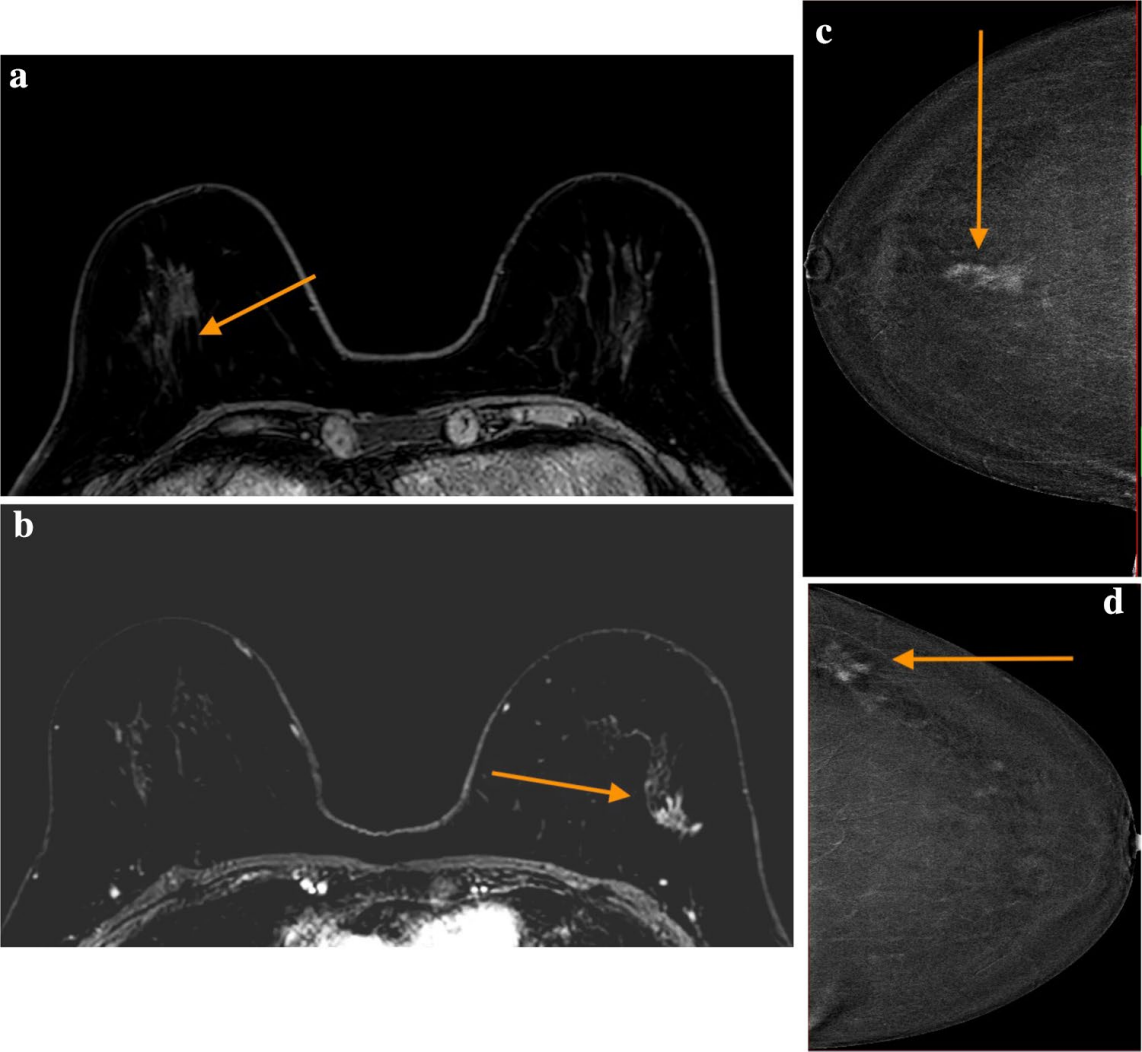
a Concordance in patient 1 MRI, right side lesion [1]. b Concordance in patient 1 MRI, left side lesion [2]. c Concordance in patient 1 CEM, right side lesion [1]. d Concordance in patient 1 CEM, left side lesion [2]
